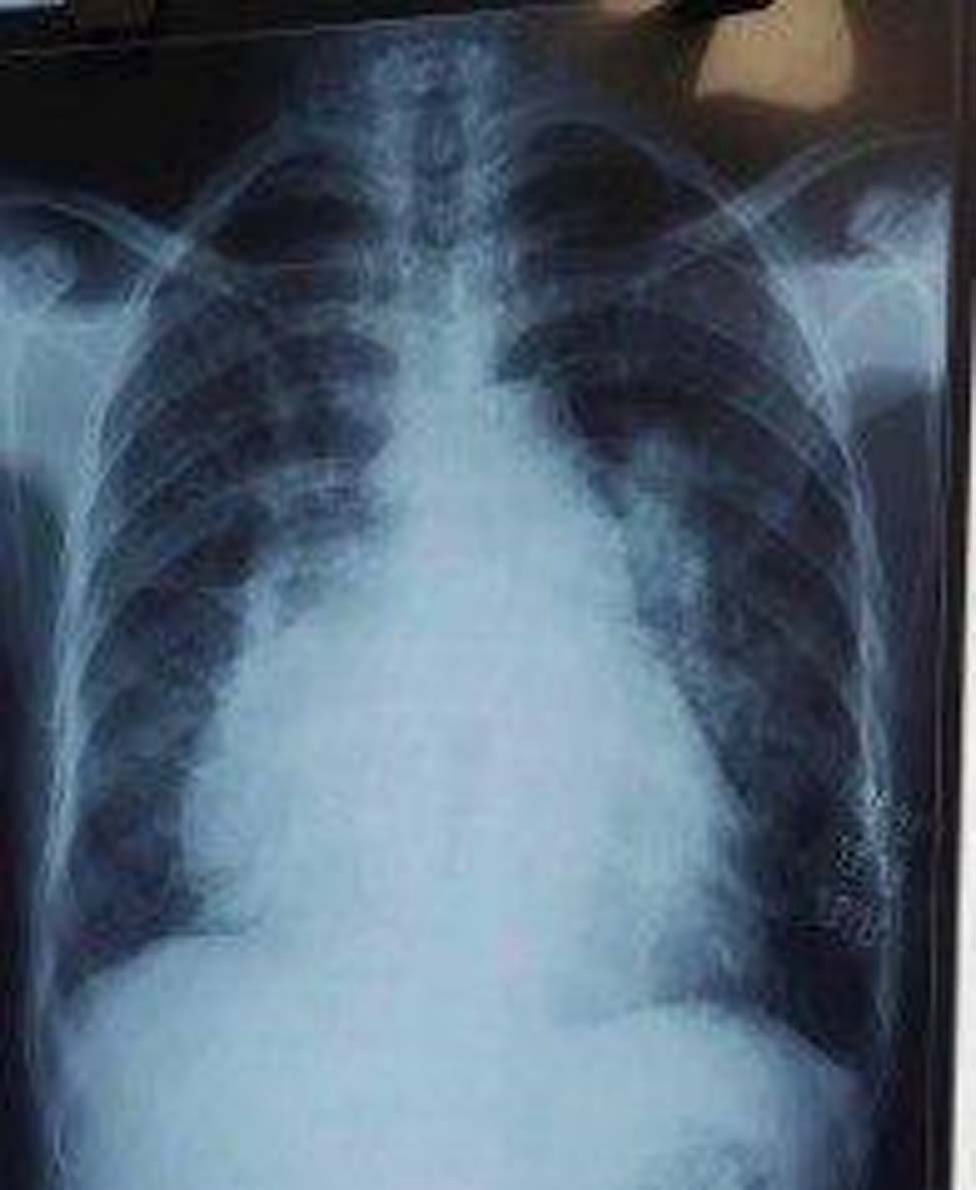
Chest X-ray showing bulging pulmonary conus, cardiomegaly with a cardiothoracic ratio of 0.7, and pulmonary plethora.
